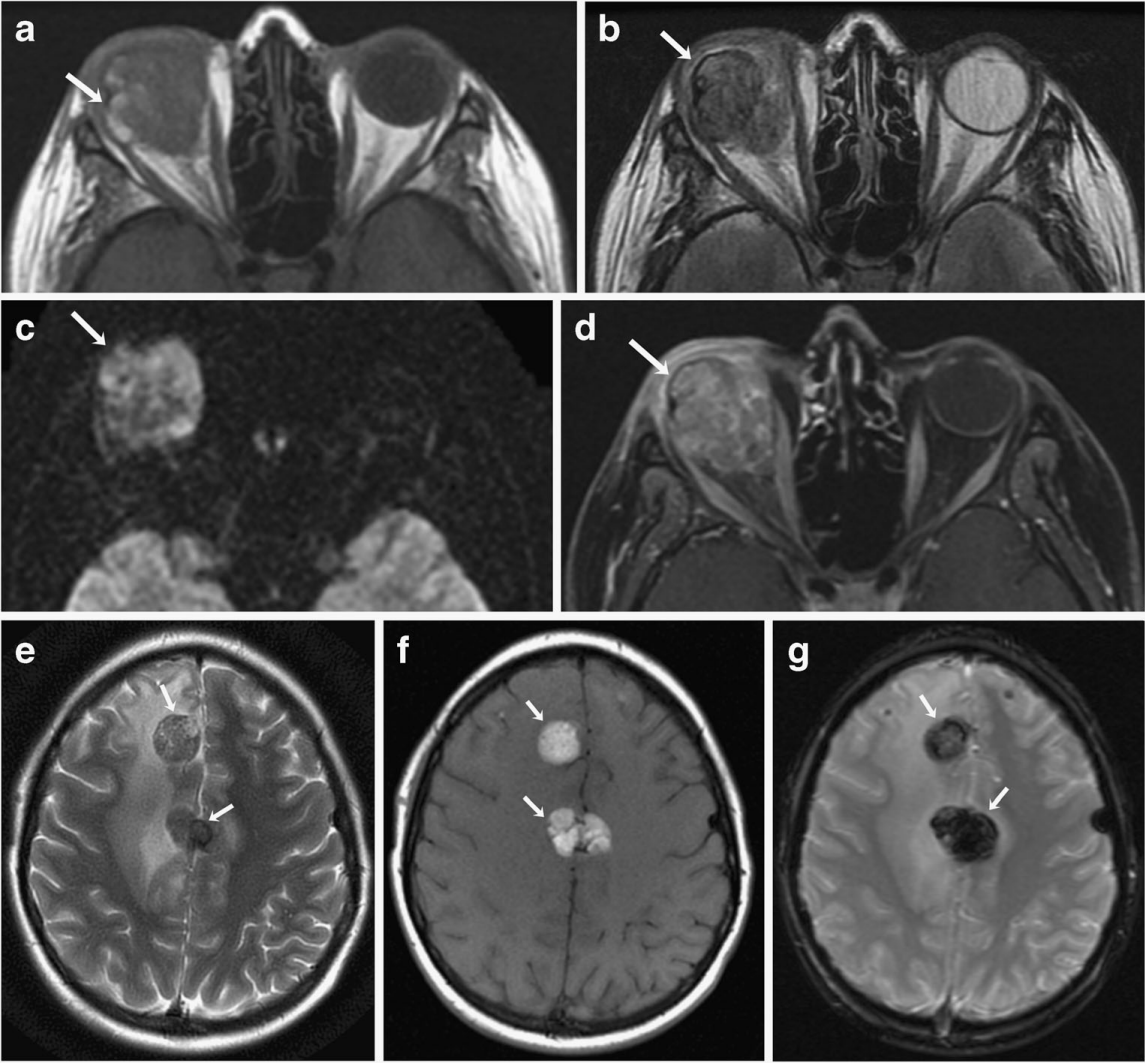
A 26-year-old male with ocular melanoma with extraocular extension and intracranial metastases. a Axial T1-weighted MR image showing a heterogeneous mass involving the right orbit with areas of T1 hyperintensity (arrow) and extraocular extension. b The mass is heterogeneously hypointense on the corresponding axial T2-weighted MR image (arrow). c On corresponding axial diffusion-weighted MRI, the mass shows restricted diffusion (arrow). d Axial contrast-enhanced T1-weighted MR image shows heterogeneous enhancement of the lesion (arrow). e Axial T2-weighted MR image shows metastatic lesions in the frontal lobes showing T2 hypointensity (arrows) with surrounding vasogenic edema. f The lesions are hyperintense on the corresponding axial T1-weighted MR image of the brain (arrows). g On the corresponding axial susceptibility-weighted (SW) MRI the lesions are hypointense (arrows) confirming a haemorrhagic component
